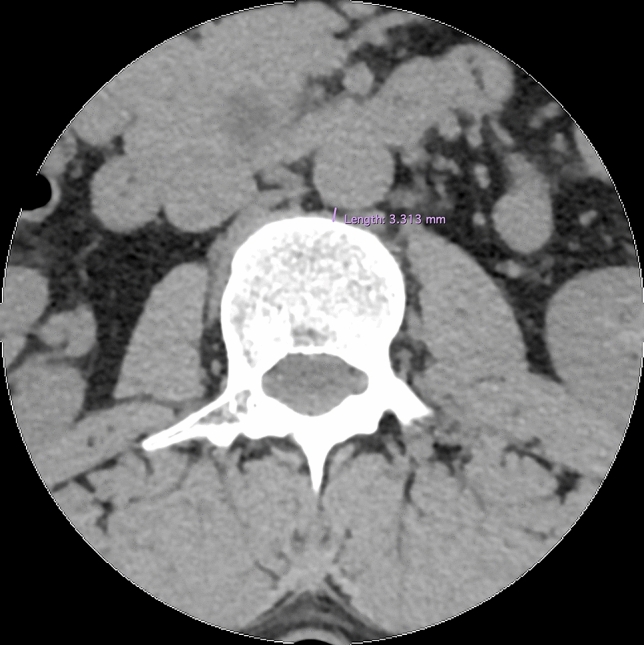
Lumbar spine computed tomography (axial) measurement of arterial-vertebra distance
